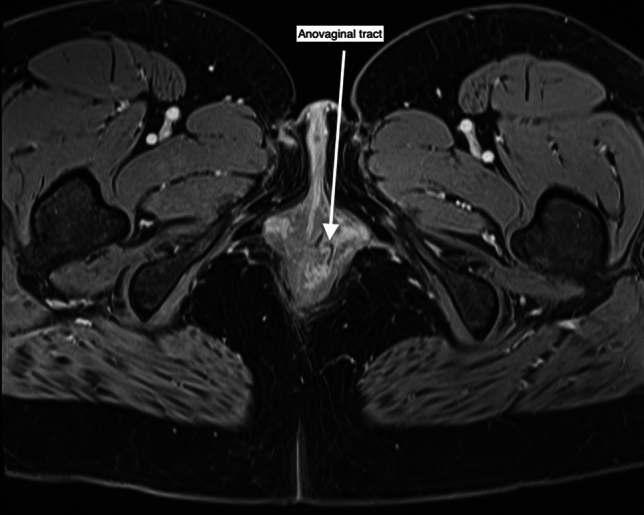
Anovaginal fistula on postcontrast MRI axial slice
